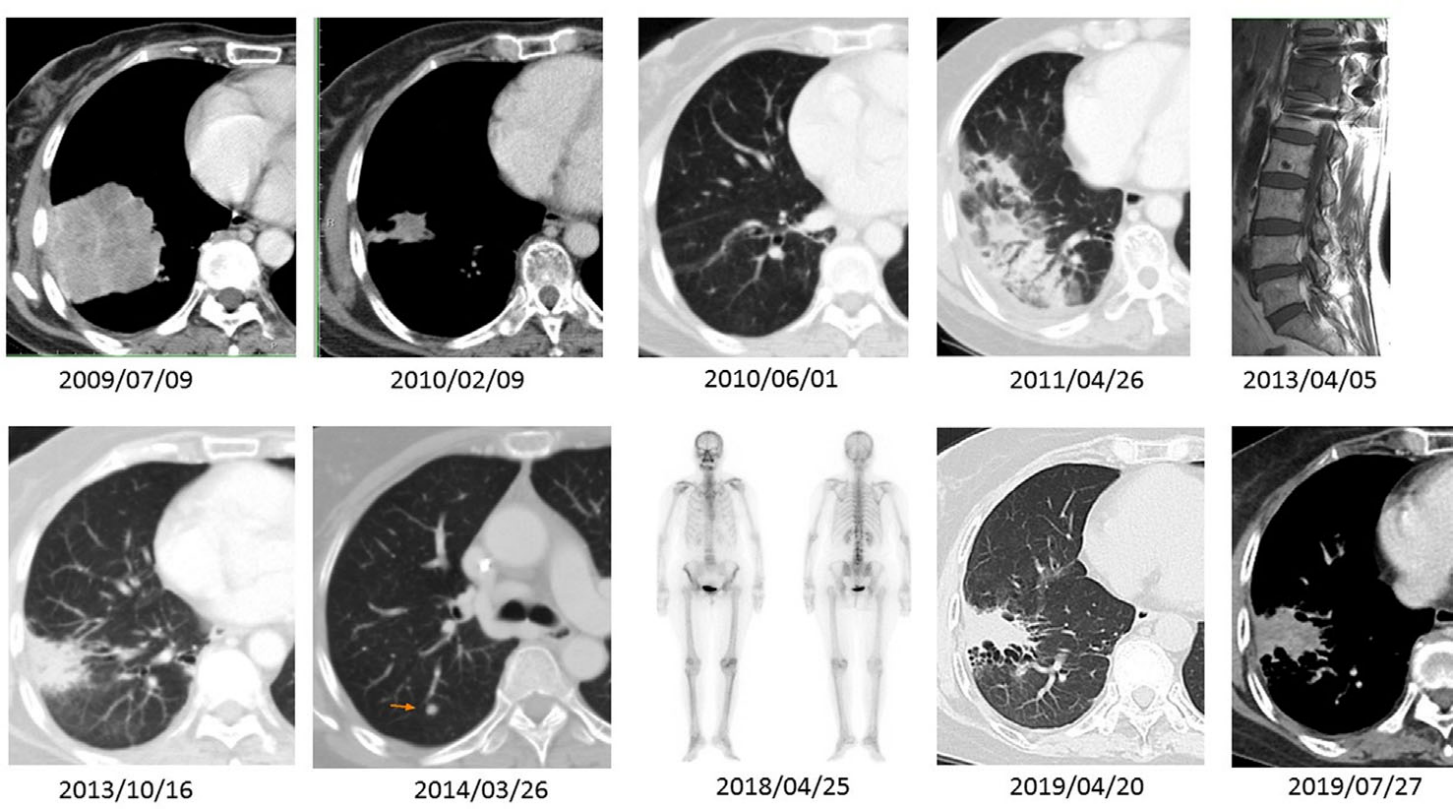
A series of imaging studies shown here are CT scans of the chest in either soft tissue or lung windows, a magnetic resonance imaging (MRI) of the spine, and a bone scan obtained spanning 10 years period. The patient had an EGFR Del-19-mutant ADC (2009/07/09). The ADC showed excellent tumor regression after five cycles of induction chemotherapy and three months of gefitinib (2010/02/09 and 2010/06/01). During effective gefitinib treatment, the patient developed an uneventful transitory pneumonitis documented on 2011/04/26. She received the same treatment until 2013/04/05, when she developed extensive-stage SCCT, causing impending spinal cord compression. There was a relapse of small cell carcinoma in the lung parenchyma (2014/03/26) after combined chemoradiotherapy with etoposide–cisplatin and prophylactic cranial irradiation. However, after lung tumor resection and second-line weekly topotecan treatment, the SCCT went into complete remission. Follow-up scans (2018/04/25 through 2019/07/27) demonstrate a quiescent bone scan and fibrotic changes at the previous ADC site.
